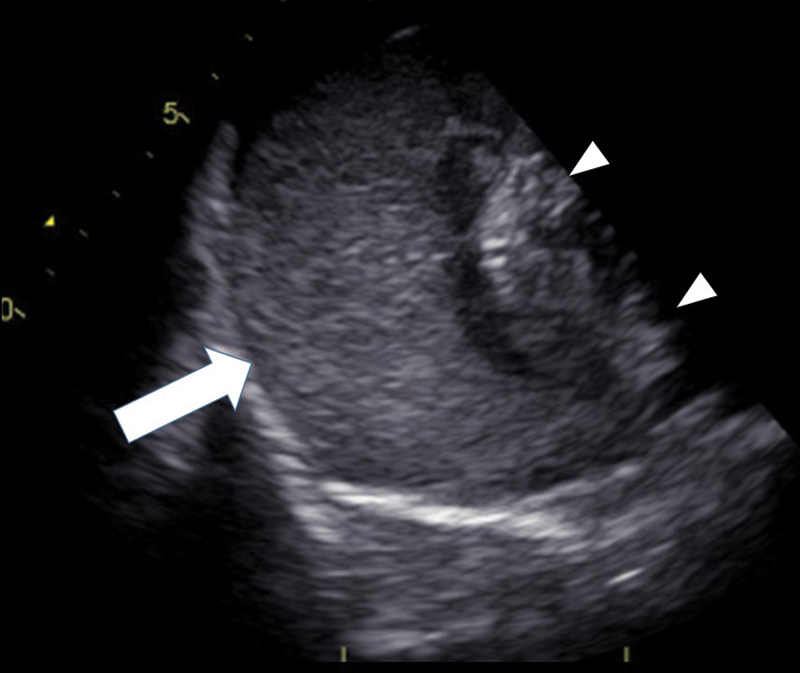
The ultrasound study revealed a high-echoic layer (arrow) surrounding the heart (arrowheads) suggesting clotting cardiac tamponade.
